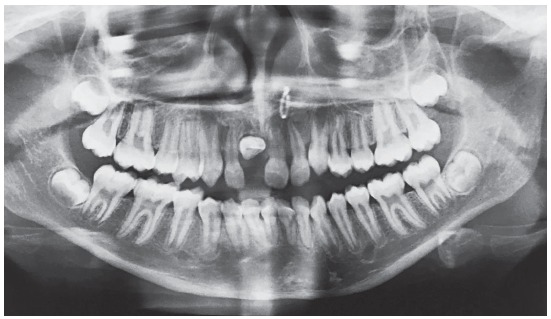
Pretreatment panoramic radiograph showing the horizontally impacted maxillary incisor.
